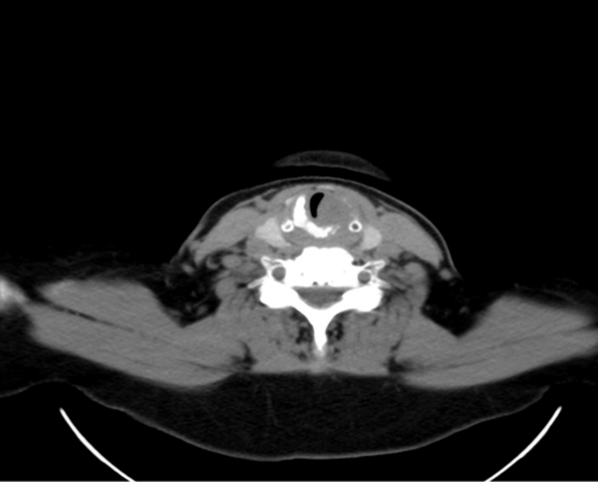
CT scan of larynx showing left vocal cord mass and destruction at left side of thyroid cartilage.
